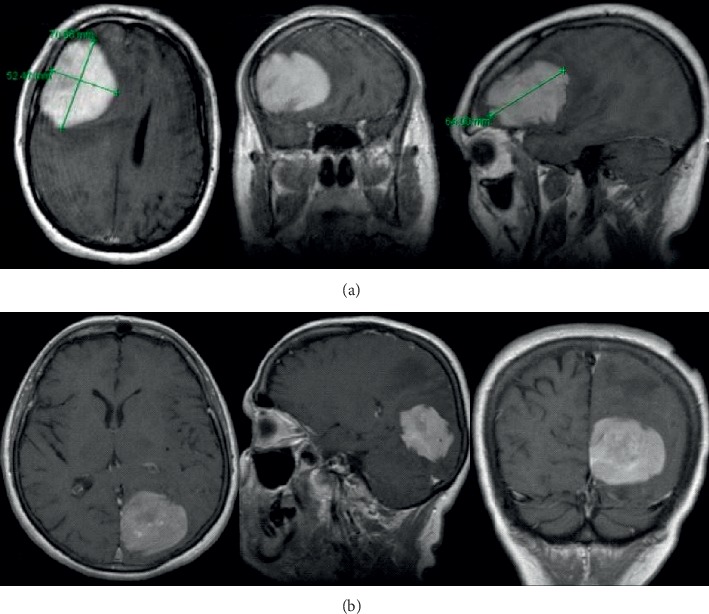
MRI study with contrast documented the presence of expansive lesions and isointense on T1-weighted, and hyperintense on T2-weighted images, characterized by an intense post-contrastographic enhancement, with dural tail, located in the first case in the right frontal region (a) and in the second case in the left occipito-parietal region (b).
